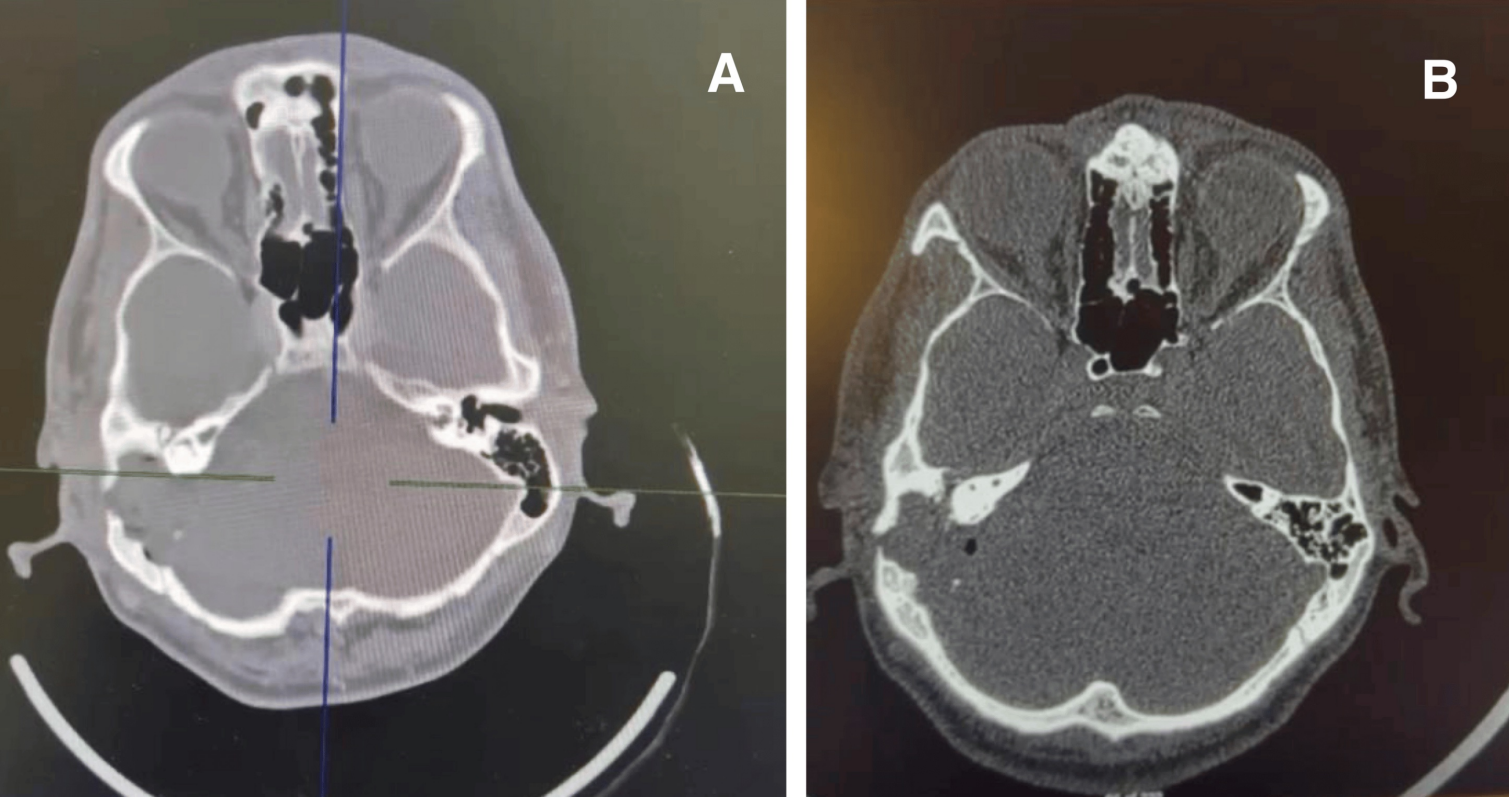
The preoperative CT scan of the right temporal bone shows a large soft tissue mass in the mastoid cavity with associated bony erosion and a focal defect in the tegmen mastoideum. A: bone window (axial cut); B: soft tissue window (axial cut)
